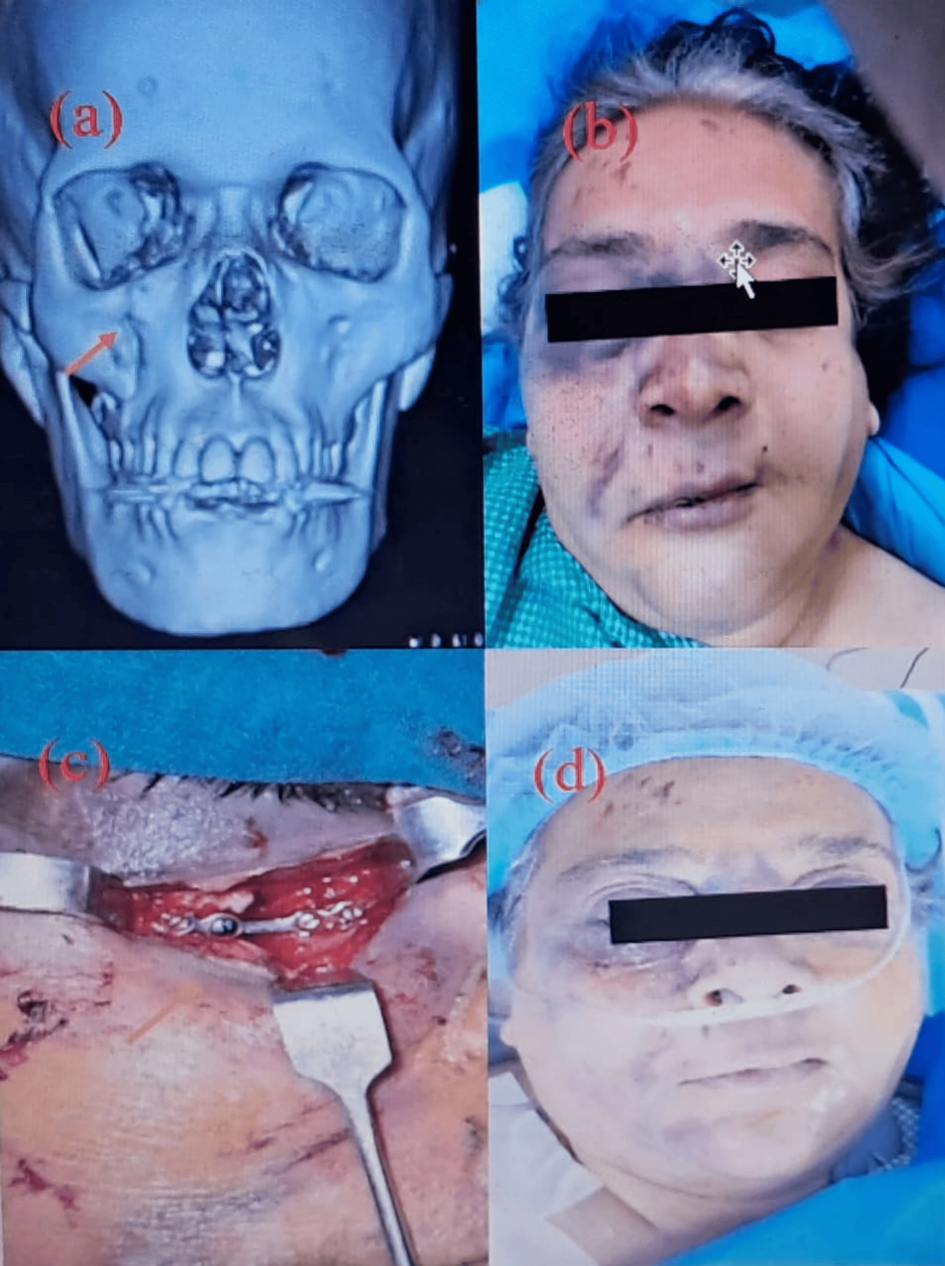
Clinical presentation and surgical management a: 3D reconstructed CT facial shows a right infraorbital fracture (red arrow); b: clinical finding showing right Raccoon's eye, subconjunctival ecchymosis; c: ORIF of right infraorbital fracture (arrow); d: postoperative picture ORIF: open reduction and internal fixation Written informed consent to include this image in an open-access article was obtained from the patient.
